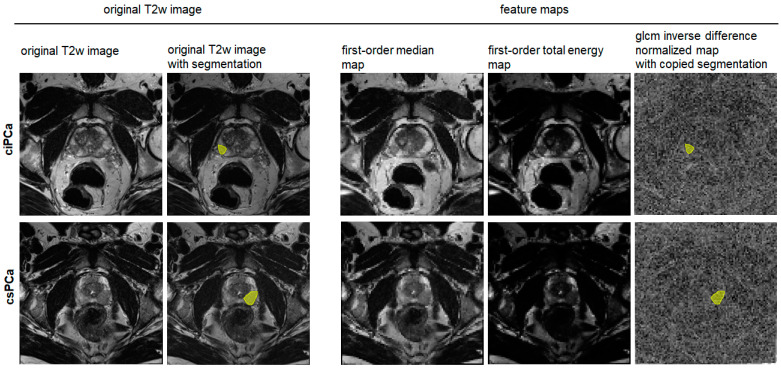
Exemplary feature maps of best-performing features in the peripheral zone. In the upper row, images of a patient with clinically insignificant prostate cancer (ciPCa, Gleason Score 3 + 3, prostate-specific antigen (PSA) density: 0.17 (ng/mL)/cm^3^) and in the lower row images of a patient with clinically significant prostate cancer (csPCa, Gleason Score 3 + 4, PSA density: 0.17 (ng/mL)/cm^3^) are shown. On the left, the original T2-weighted axial image and the segmented target lesion marked with a volume of interest (VOI, yellow) are displayed. On the right, the parametric feature maps of the first-order median, first-order total energy, and glcm inverse difference normalized are shown. The map-derived features first-order median and first-order total energy revealed excellent diagnostic performance in differentiating csPCa from non-csPCa (no PCa and clinically insignificant PCa). VOIs were copied based on software in the maps, as shown for the glcm inverse difference normalized map, to extract feature quantity directly.
